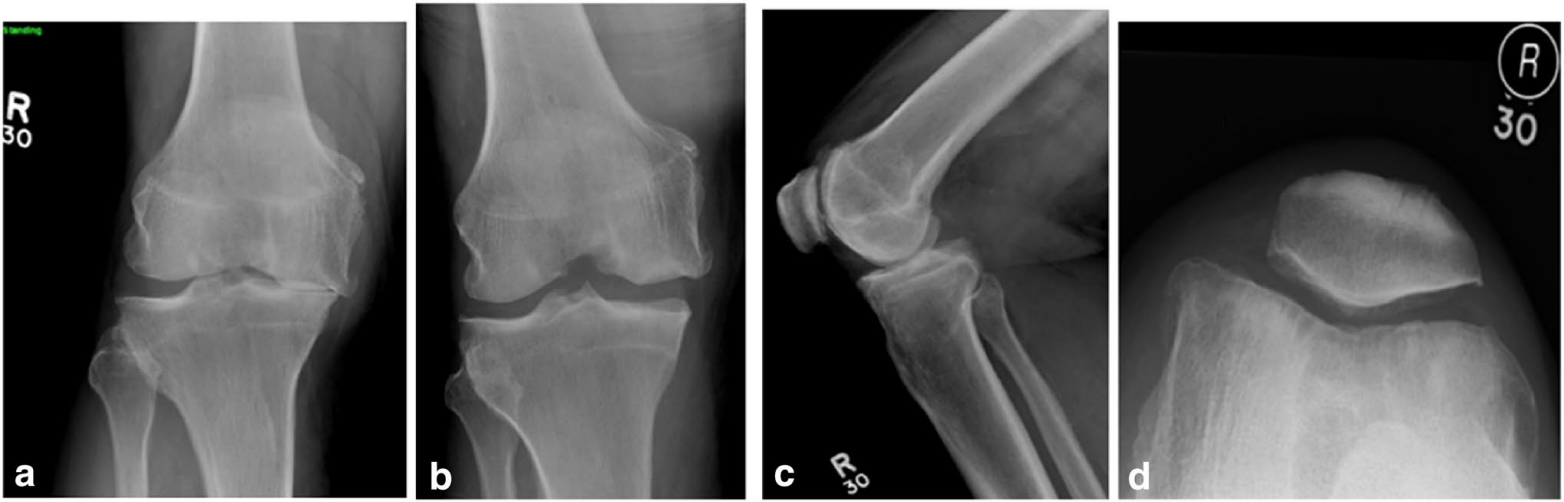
X-ray showed severe medial compartment arthritis with bone loss. a Rosenberg at 30° flexion, b Valgus stress, c lateral, d Skyline
